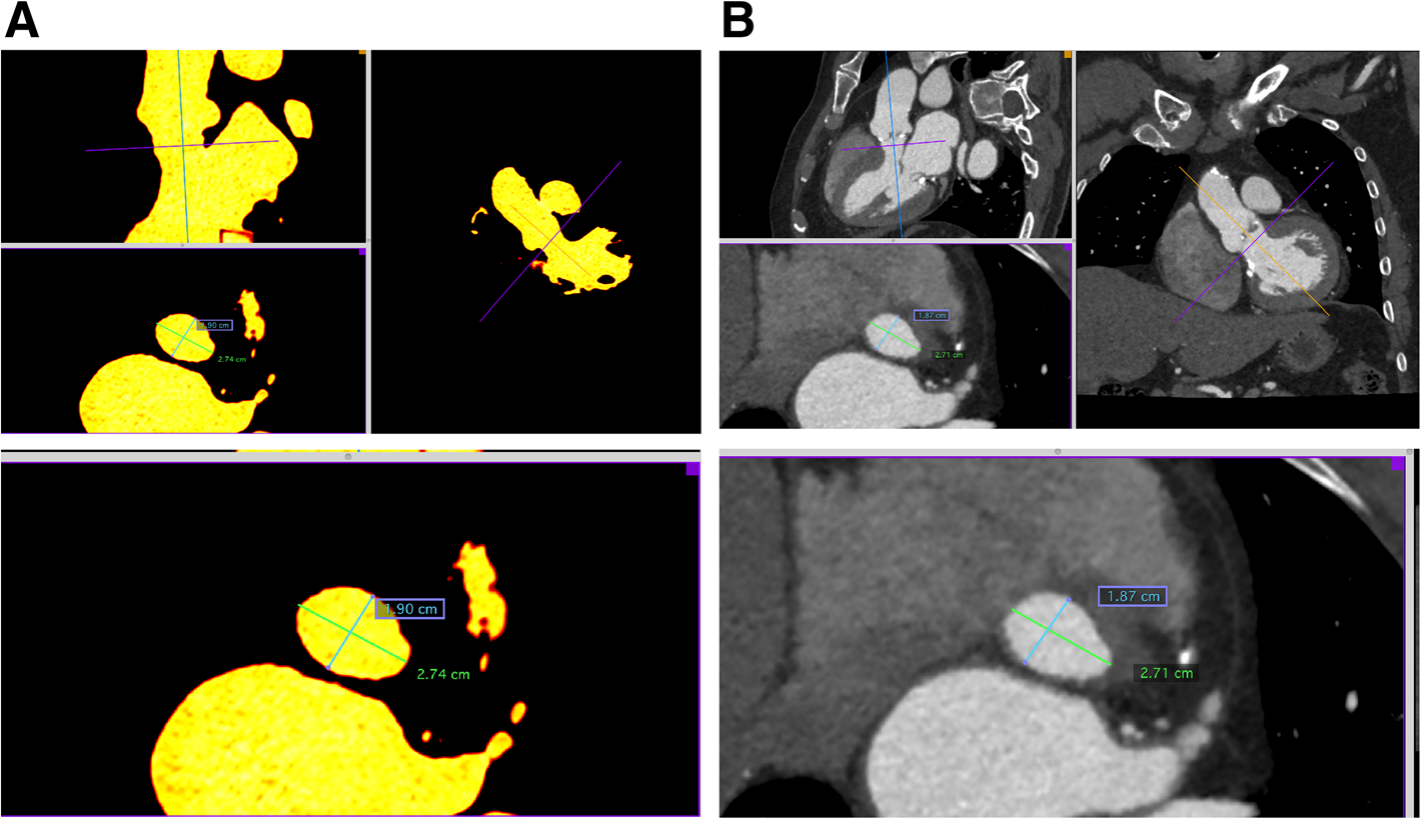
Quantitative measurements of a 3D printed model by CT. Measurements of critical features (e.g., left ventricular outflow tract just inferior to aortic annulus) were made on final 3D printed model DICOM images (a, in yellow) and checked against the source patient DICOM data (b)
